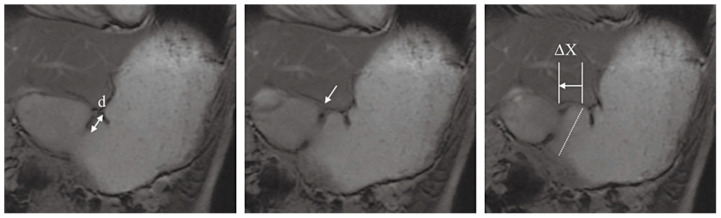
Magnetic resonance imaging of gastric peristalsis; arrows: antral contraction waves; d: the respective deepness of the wave; ΔX: the travel distance of the wave in 20 s; the figure is reproduced from [11] with permission from John Wiley & Sons, Inc.
